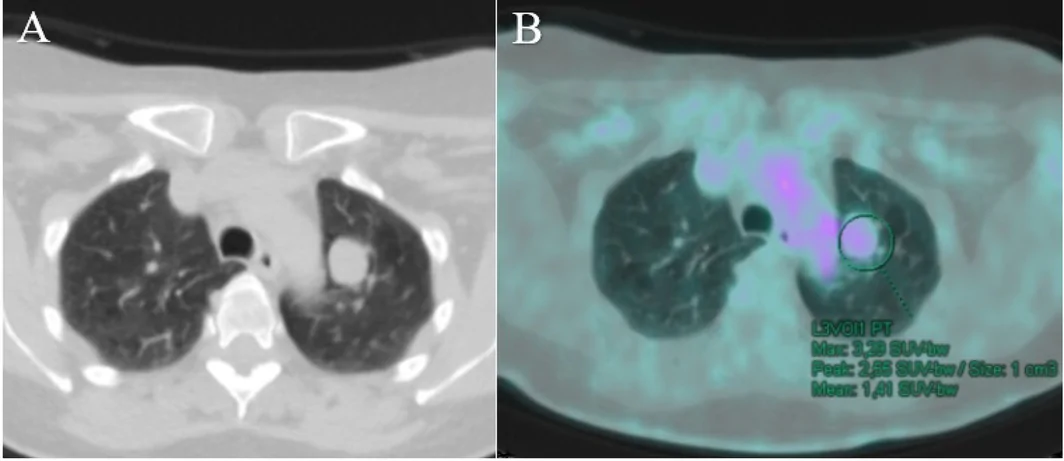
Section of the tumor on CT (A); section of the tumor on PET (B).
